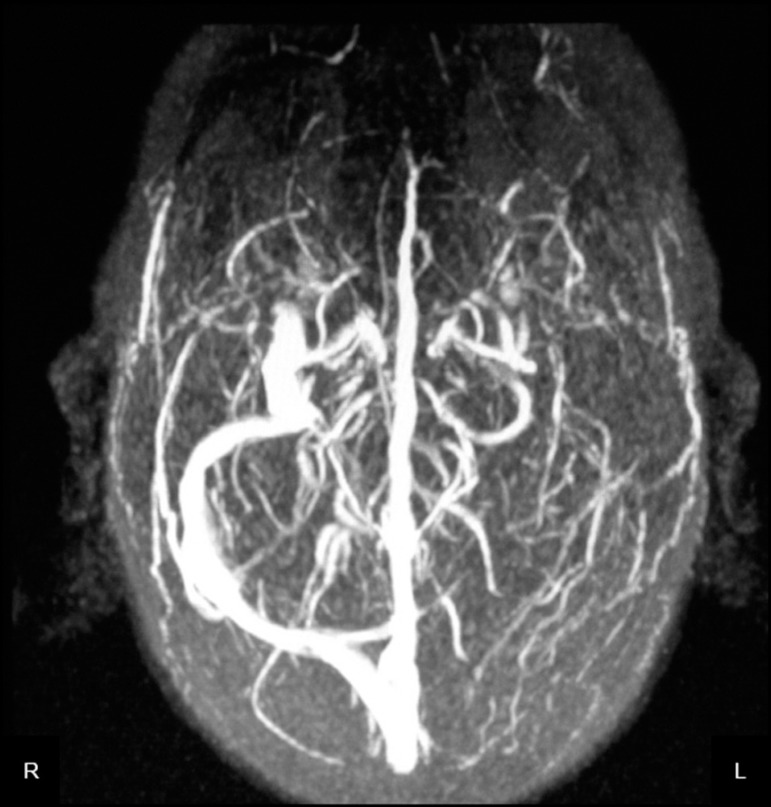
Venous brain MRI performed at second day with filling failure from left
